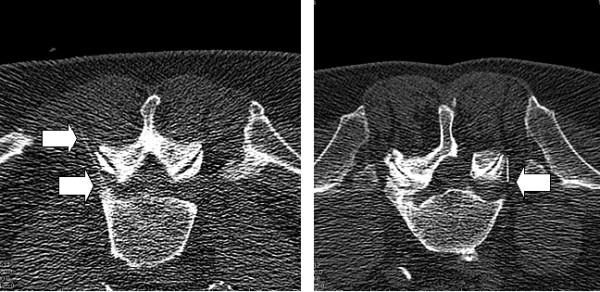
CT-guided nerve root L5- injections. CT-guided periradicular injections at the nerve root L5 in low-dose mode in two male patients. Note the acceptable visibility of the needle tip (arrow) in both interventions, even in reduced contrast. The right image presents a status post hemilaminectomy.
